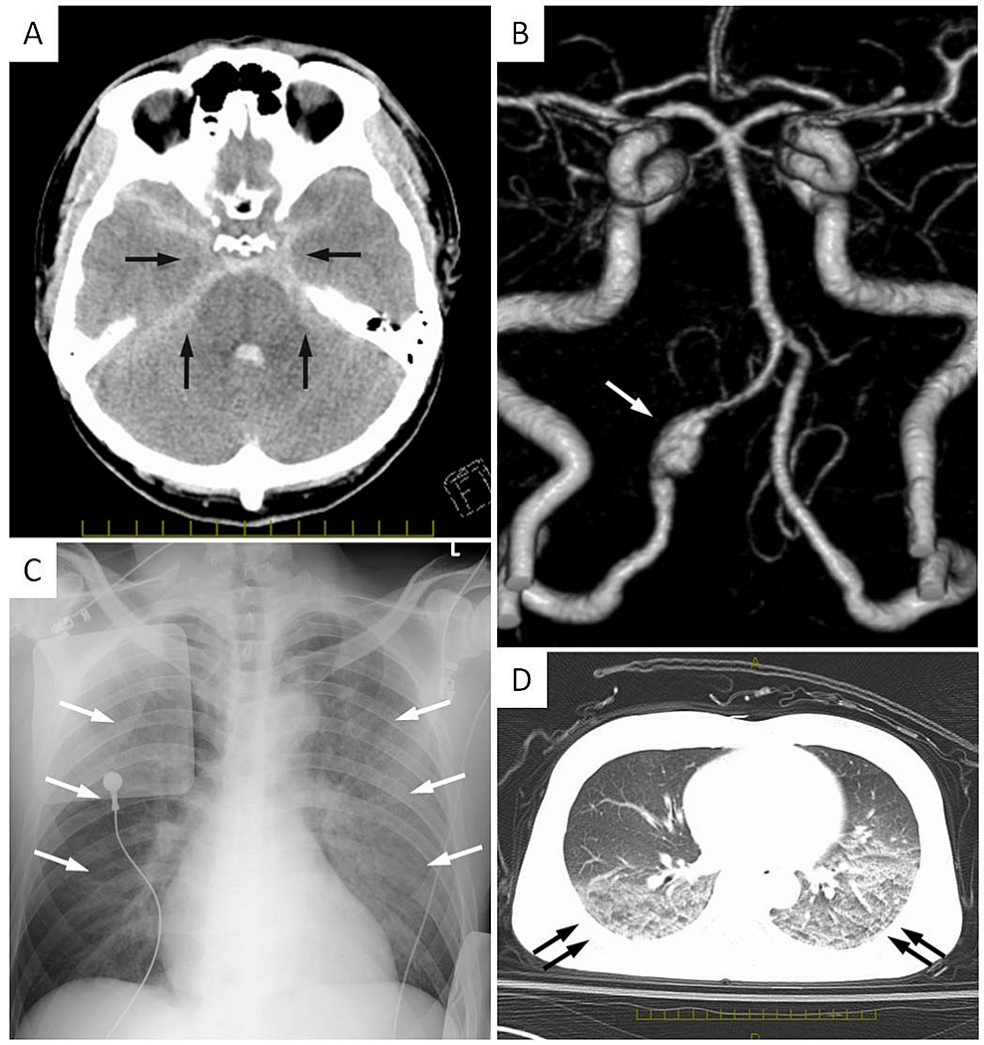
Initial radiographic images. (A) Head CT shows diffused subarachnoid hemorrhage in the basal cistern (arrows). (B) Three-dimensional CT angiogram shows the dilation of the right vertebral artery, indicating dissecting aneurysm (arrow). (C) Chest X-ray shows decreased permeability of the lung field (arrows). (D) Chest CT shows pulmonary infiltration in bilateral lung fields, indicating pulmonary edema (double arrows).
